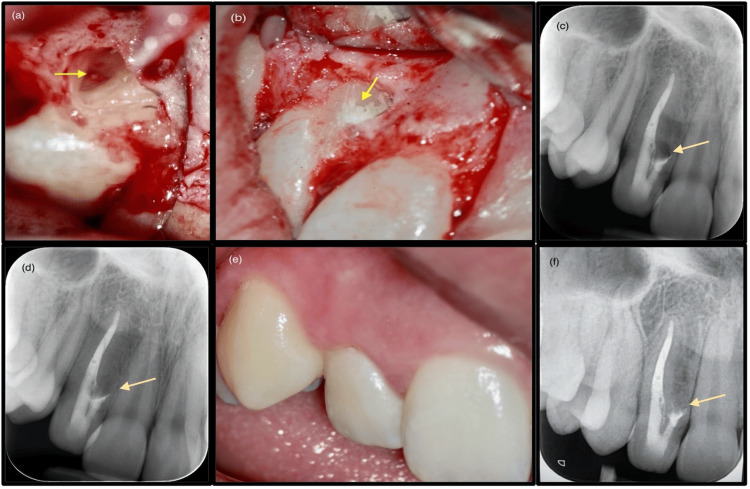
(a) Clinical photograph of tooth #12 after flap reflection, showing incomplete radicular formation near the cementoenamel junction and the mid-radicular defect with loss of the buccal plate (arrow) (×10). (b) Clinical photograph after resection and placement of MTA retrograde filling into the DI defect (arrow) (×10). (c) Immediate postoperative radiograph showing MTA retrofill/repair placement (arrow). (d) Twelve-month postoperative radiograph demonstrating reduction of the radiolucency (arrow). (e) Intraoral photograph at 12-month recall showing soft tissue healing and absence of a sinus tract. (f) Radiograph at three years and 10 months demonstrating complete osseous repair and mineralization of the defect (arrow). DI, dens invaginatus; MTA, mineral trioxide aggregate
